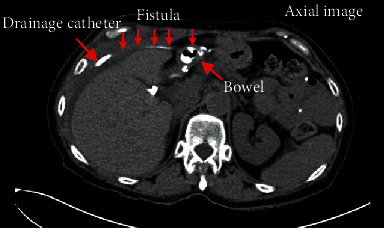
CT fistulogram showing an enterprising-atmospheric fistula with gastric remnant. Figure provided by Louis Maurice Morel-Ovalle, MD, DABR, and Raj Bant.
